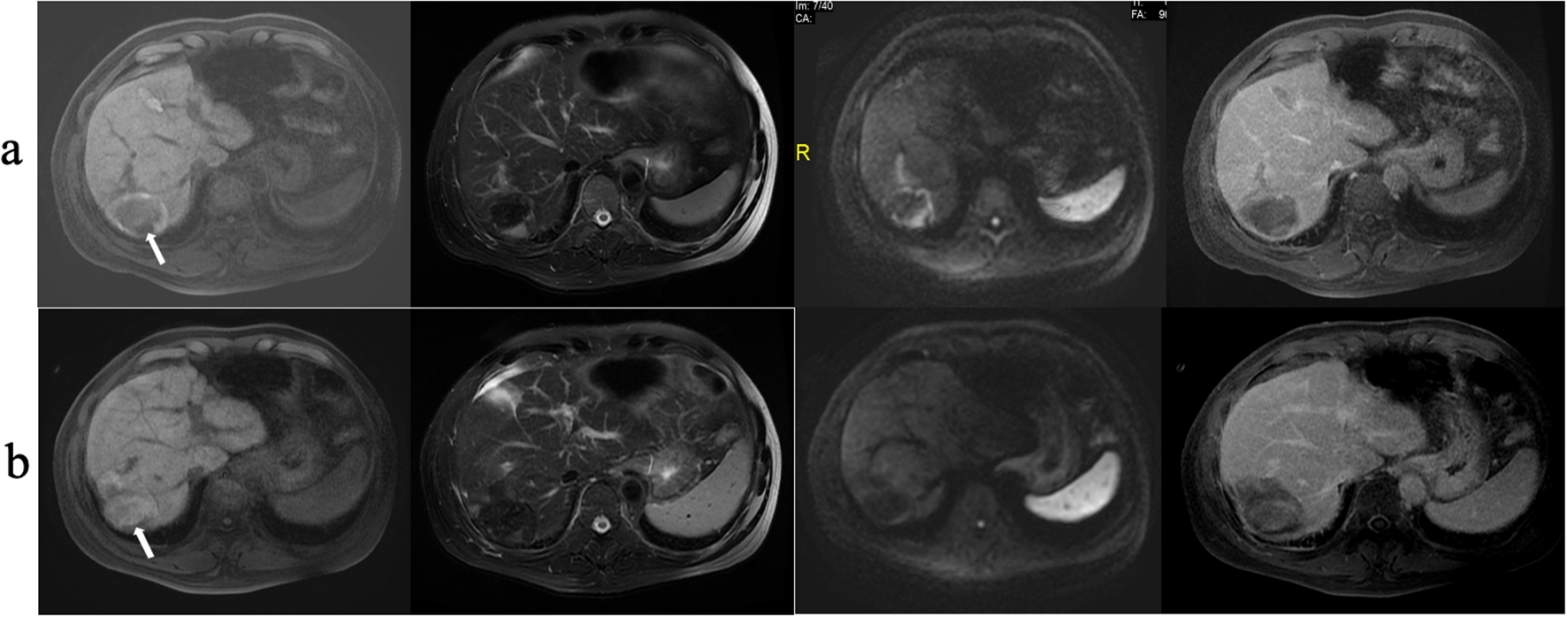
RFA treatment of HCC. (A) MRI shows a HCC lesion 4.9 cm in diameter; (B) MRI scanning at 12 months after RFA treatment shows that the ablated area had shrunk significantly.
